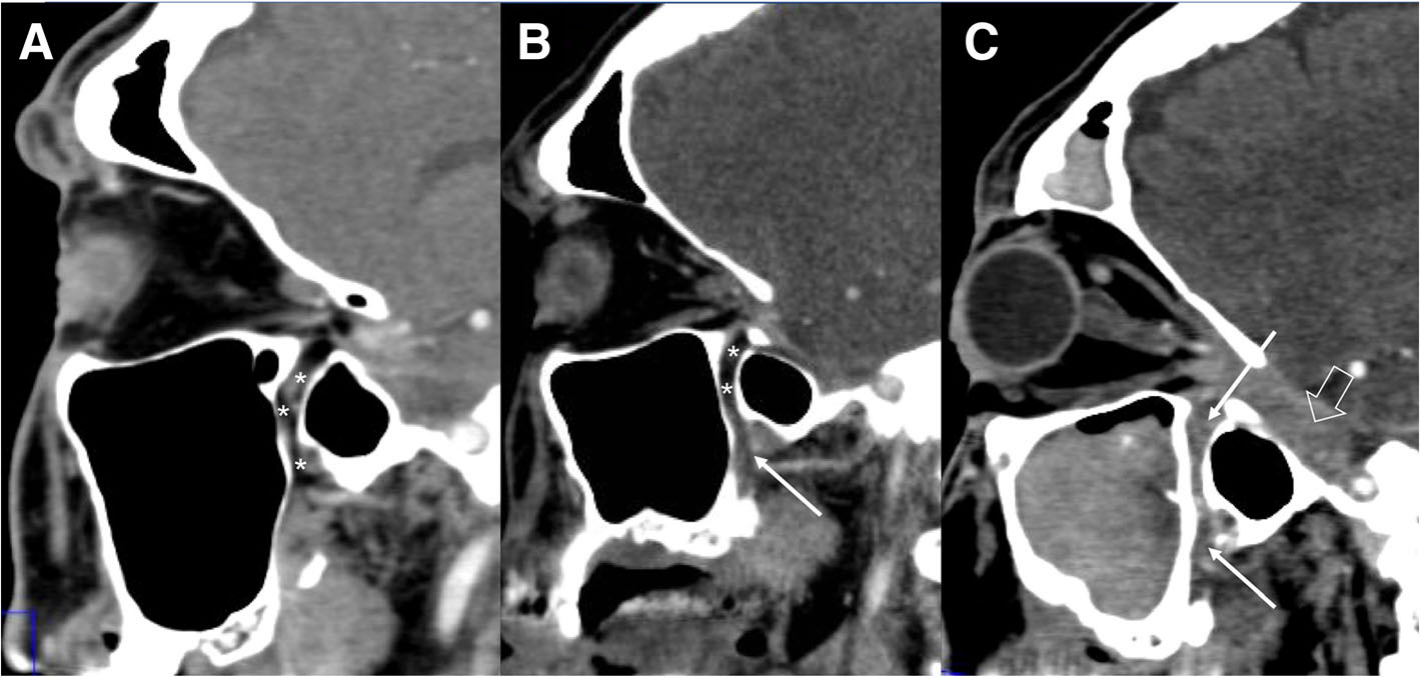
Perineural spread of tumor. Patient with squamous carcinoma of the retromolar trigone. CT sagittal images at 0 (a), 6 (b), and 12 (c) months follow-up. Note the progressive infiltration (arrows) of the pterygopalatine fossa (asterisks) with obliteration of the normal fatty tissue. In (c), it is evident the invasion of Meckel’s cave (empty arrow)
